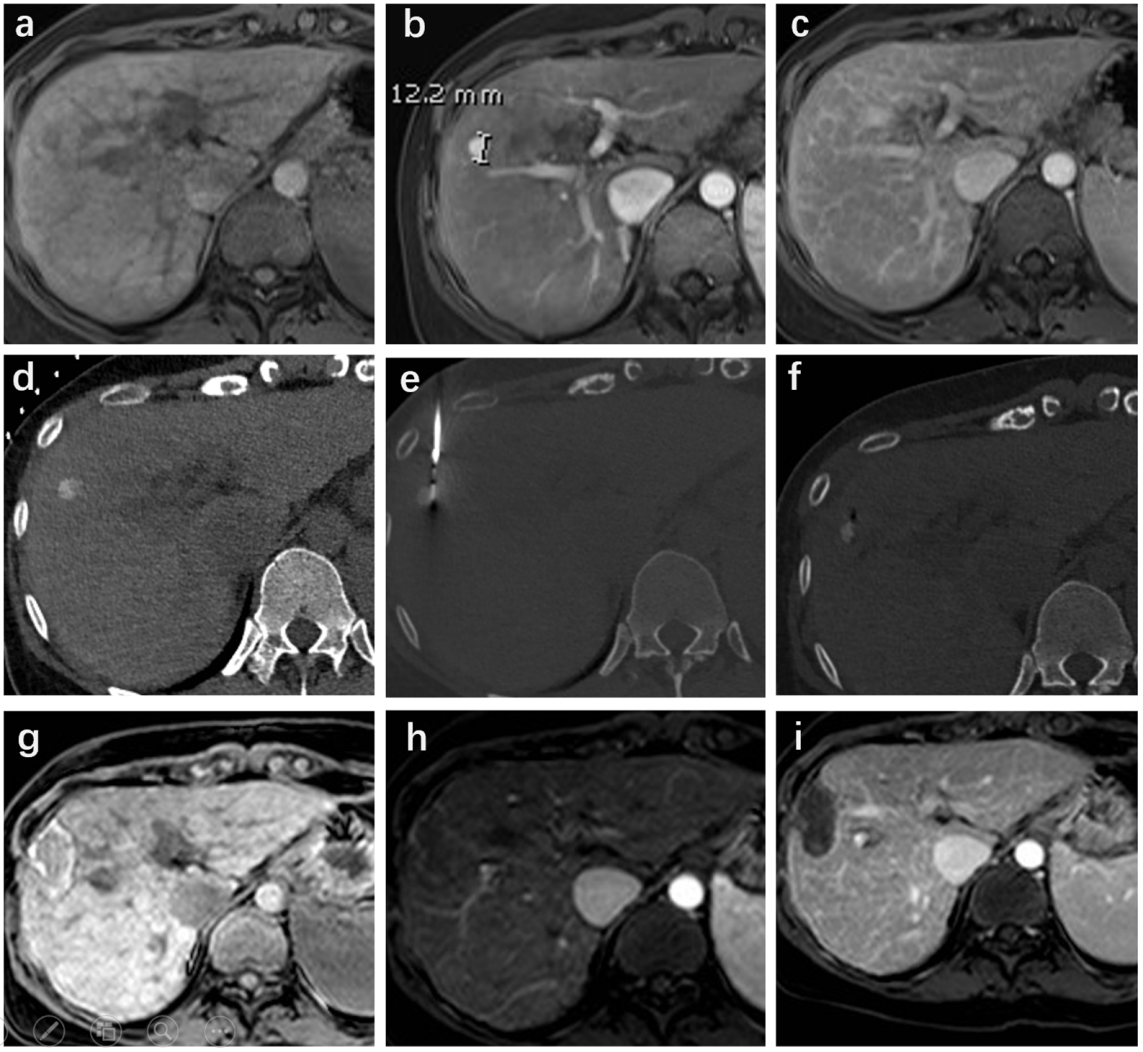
A 63-year-old female with hepatitis B was diagnosed with Li-Rads 5 hepatocellular carcinoma (HCC) nodular in segment VIII. The pre-ablation magnetic resonance imaging (MRI) showed the lesion located in the right lobe with non-rim enhancement in the arterial phase (maximum diameter of 12 mm) and non-peripheral washout with an enhancing capsule in the delayed phase (A-C). The lineament of the HCC lesion with iodized oil retention was well depicted in the CT scan (D). CT-guided microwave ablation (MWA) was performed under local anaesthesia plus sedation (E, F). Follow-up MRI was performed four weeks after ablation and showed a complete response (G-I).
